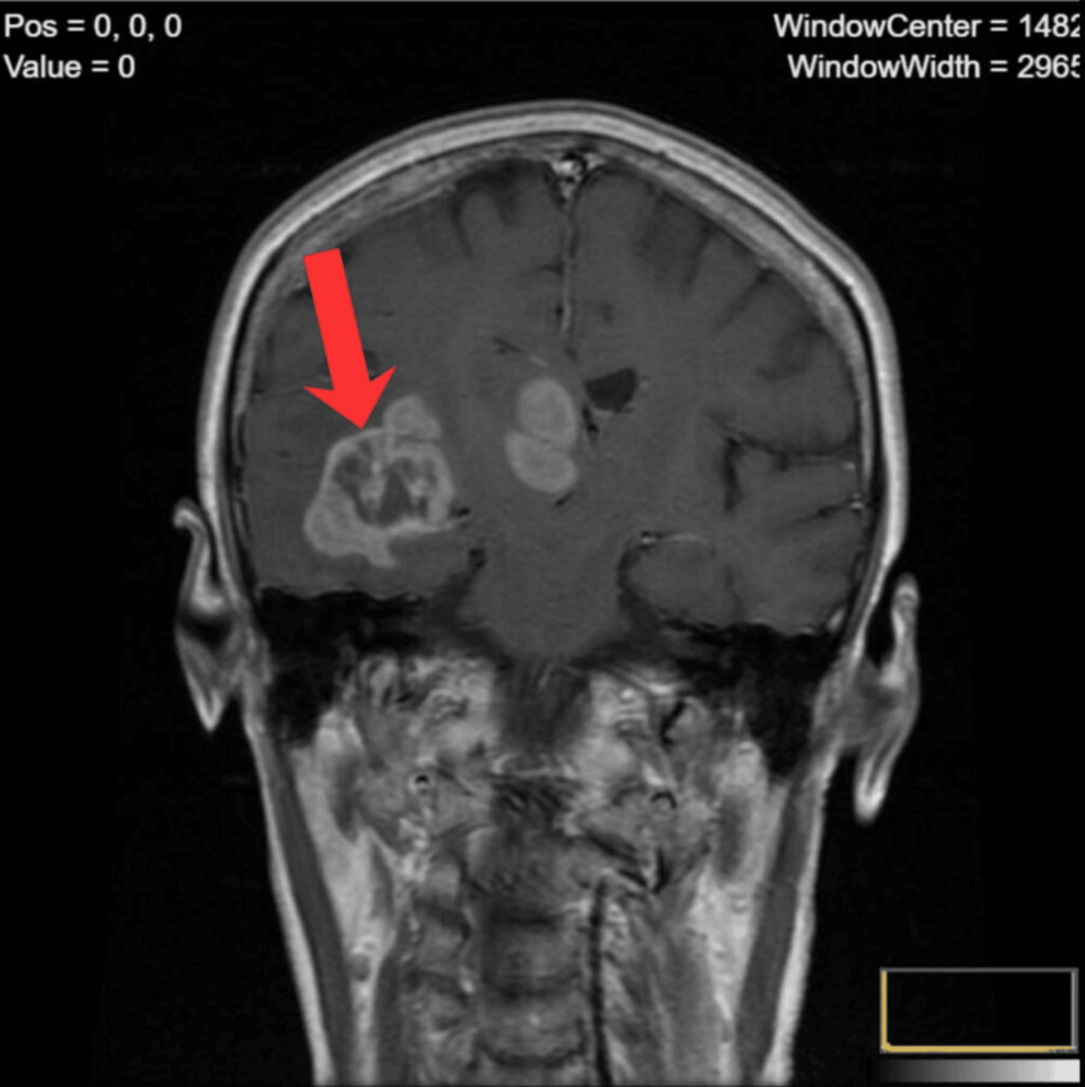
Coronal contrast-enhanced MRI of a right temporal lobe mass Coronal contrast-enhanced MRI demonstrating a large irregularly enhancing mass within the right temporal lobe, accompanied by a second adjacent enhancing lesion. The dominant tumor exhibits ring-like enhancement with central necrosis and surrounding vasogenic edema, producing significant mass effect on midline structures.
